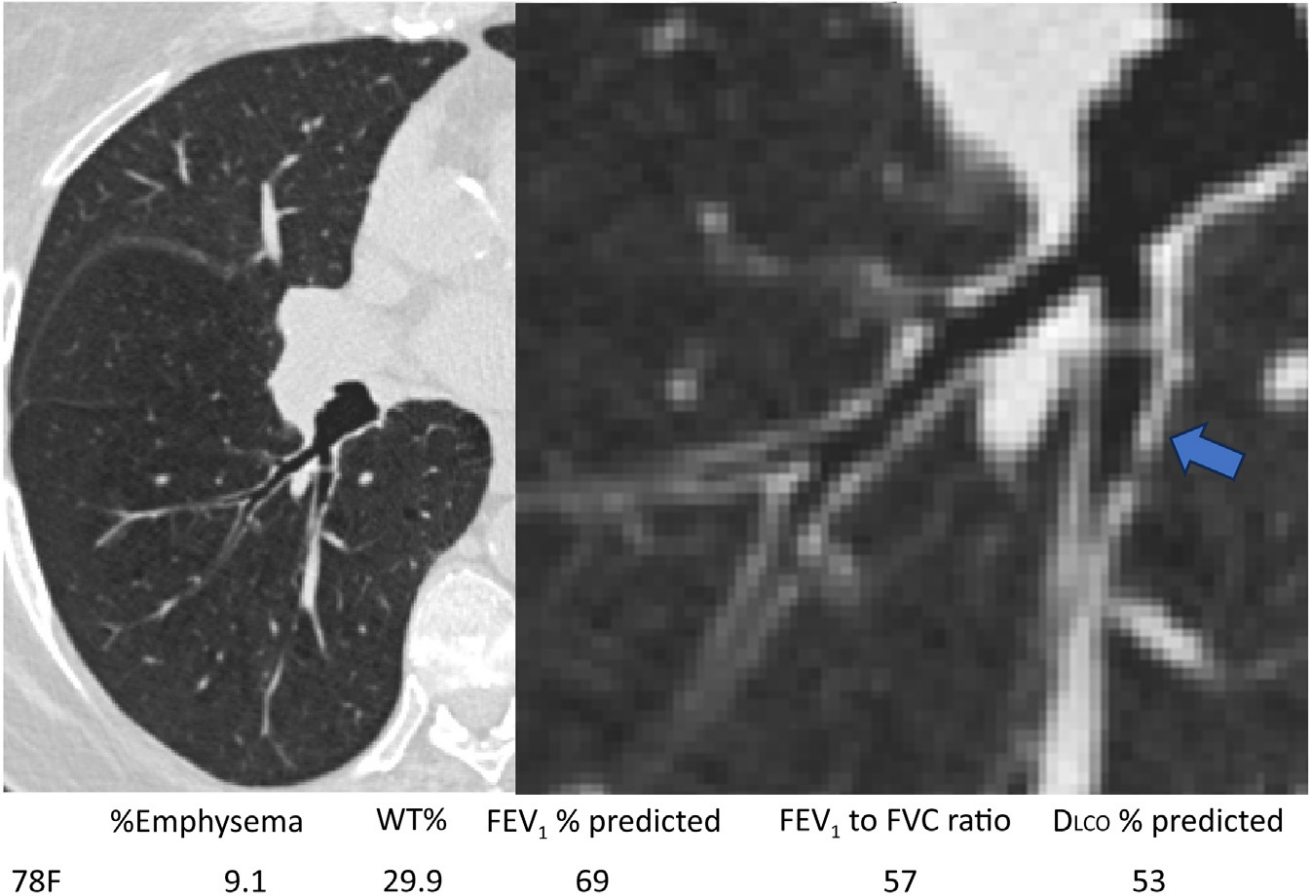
CT scans of airways of representative patients with bronchial wall thickening denoted by blue arrow. Below the figure, quantitative CT imaging variables also are included (%Emphysema and WT%), in addition to pulmonary function testing values (FEV_1_, FEV_1_ to FVC ratio, and Dlco % predicted). Dlco = diffusing capacity of the lungs for carbon monoxide; %Emphysema = percentage of emphysema; WT% = wall thickness percentage.
